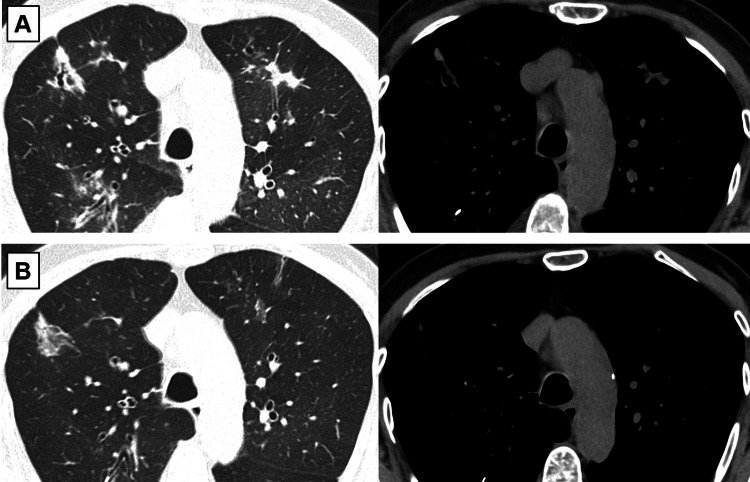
Computed tomography images of the chest (A) during symptom flare-ups two months after the initiation of benralizumab therapy and (B) when imaging findings markedly improved after the administration of dupilumab
